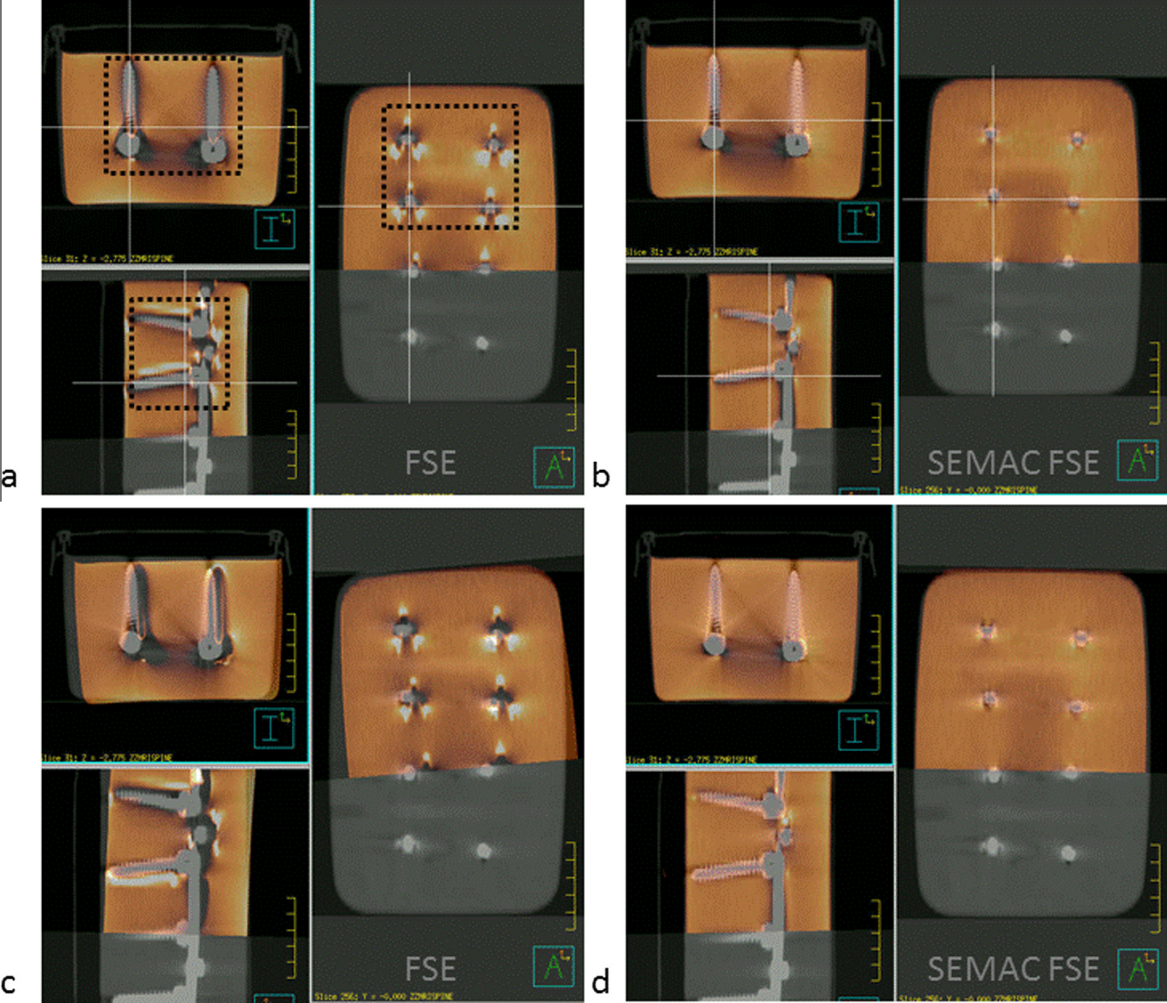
Conventional MR FSE (left) and MR SEMAC FSE images (right), overlaid on CT. The MR images cover a smaller volume. (a) and (b) show the best possible co-registration (gold standard), which uses the position of the gel-filled plastic box as a reference; (c) and (d) show the best automatic co-registration (Pinnacle, Philips), which is clearly disturbed by structured artifacts.
